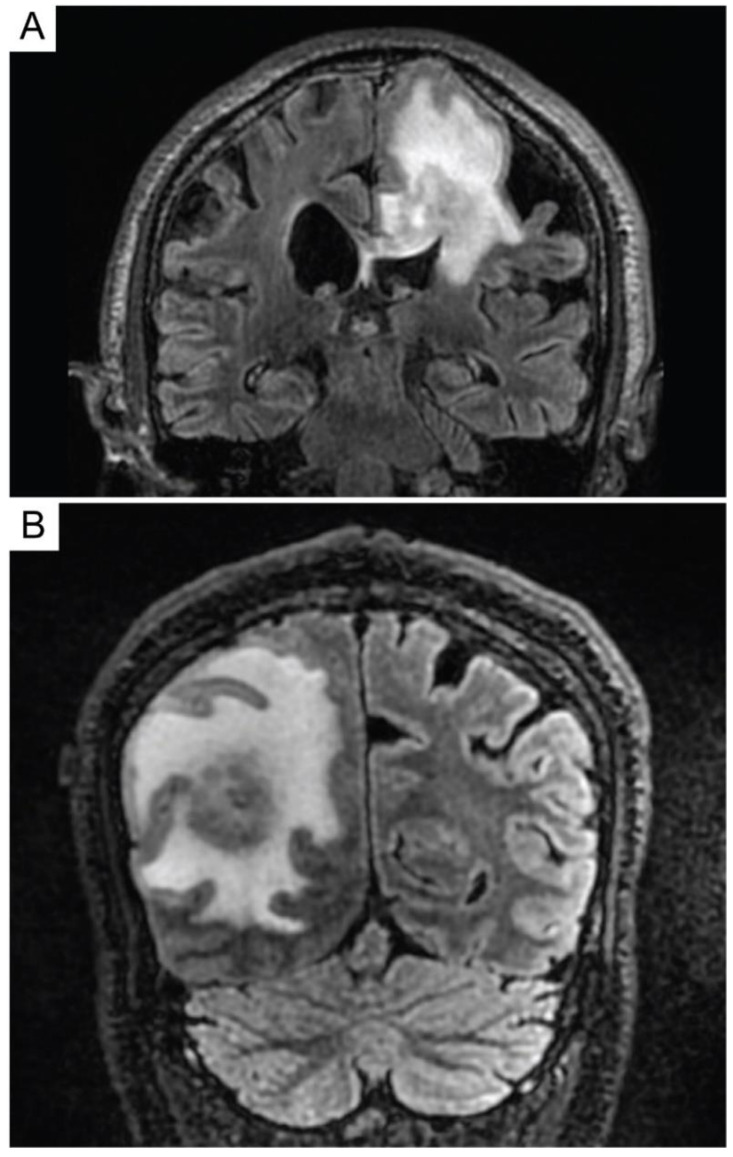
MRI images of a primary central nervous system lymphoma. T2-weighted-Fluid-Attenuated Inversion Recovery (T2-FLAIR) images of patients with primary central nervous system lymphoma that demonstrate T2 hyperintense lesions with increased signal within the surrounding parenchyma. Lesions may mimic primary glial tumors, and when involving the corpus callosum may overlap in appearance with glioblastoma (A). Patients may present with a solitary mass with significant peritumoral edema (B) or also present with multifocal intracranial disease.
